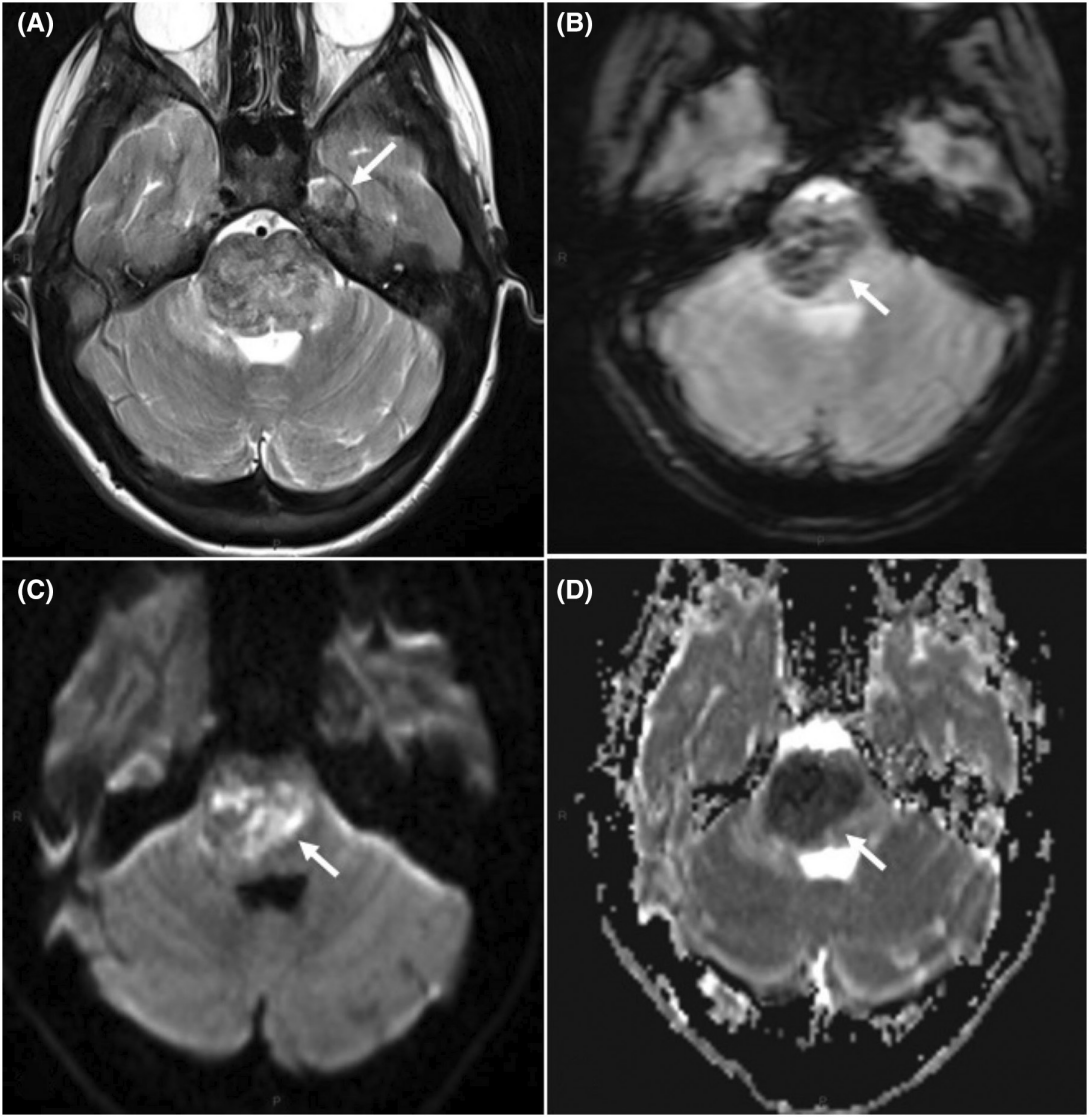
Follow‐up MRI two weeks later. Four axial transverse slices in similar slice location. (A) T2‐W view shows the extension of the parenchymal damage to the whole Pons with heterogeneous mosaicism of hyper/hypointense foci. Schwannoma of the left Vth cranial nerve (arrow). (B) gradient‐echo T2*‐W view shows strongly hypointense susceptibility artifacts revealing the presence of deoxyhemoglobin. (C) DW view shows hyperintense areas within the lesion (arrow) featuring cytotoxic edema due to ischemia. (D) ADC‐mapped view confirms a strong ischemia‐related decrease in apparent diffusion coefficient (ADC) values.
